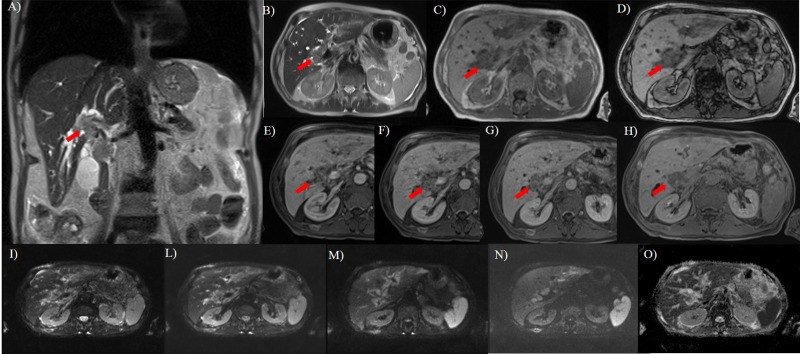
Fifty years old man with rectal cancer. In A (T2-W HASTE coronal plane) and B (T2-W HASTE axial plane), the lesion (arrow) appears as a single periductal hyperintense mass extending to the bifurcations of both right and left hepatic ducts. In C (axial T1-W in phase) and D (axial T1-W out phase) the lesion (arrow) show hypointense signal. During arterial (E), portal (F), equilibrium (G) phase of contrast study the lesion has a progressive contrast enhancement while during hepatobiliary phase (H), is hypointense. DWI sequences: In I *b*0 s/mm^2^, in L *b*50 s/mm^2^, in M *b*400 s/mm^2^, in N *b*800 s/mm^2^ and in O ADC map. The tumor shows restricted diffusion.
